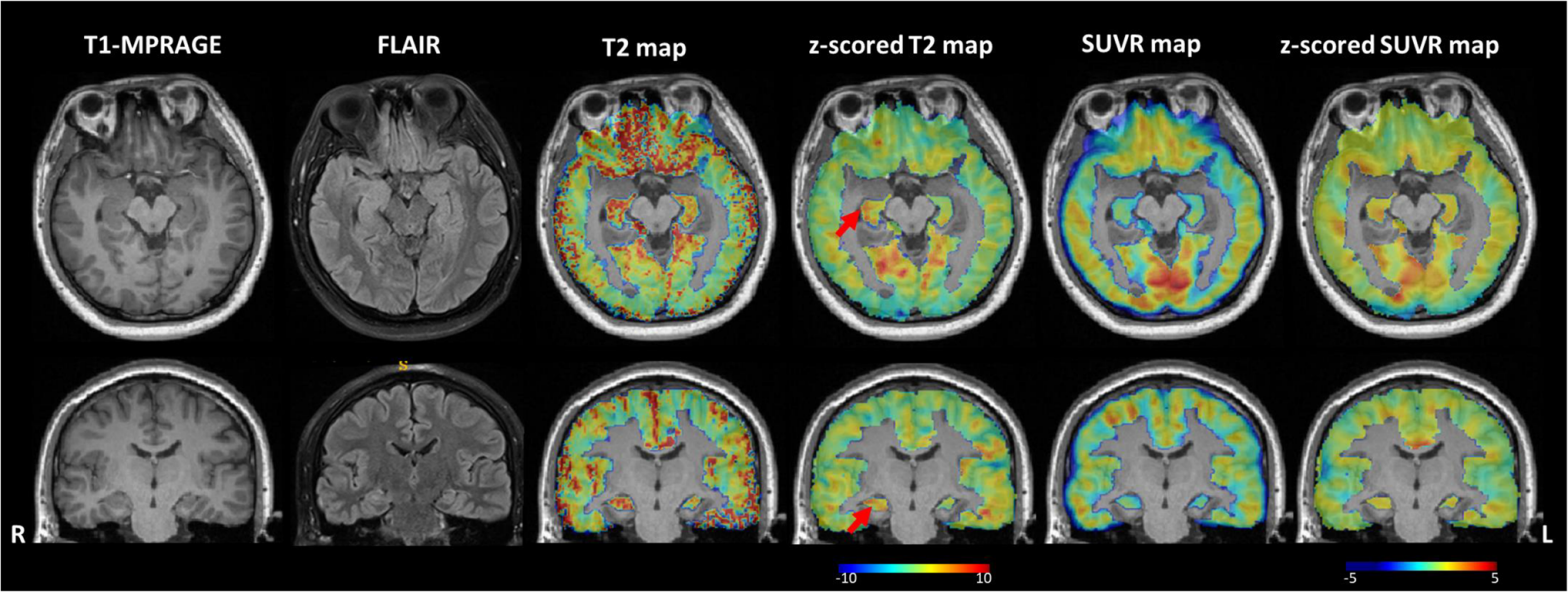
T1-weighted (MPRAGE), FLAIR, PET SUVR, and T2 maps and the *z*-scored maps of a typical MRI-negative RTLE patient, #9. The T1-weighted image, FLAIR image, SUVR, and *z*-score SUVR map have normal findings. T2 map and *z*-scored T2 map show a well-defined increased T2 signal in the right hippocampus and parahippocampus compared with the left hippocampus. SEEG findings indicated the seizure onset originated from the right hippocampus. After right temporal lobe resection, the patient had a follow-up time longer than 1 year, showing Engel class I outcome
